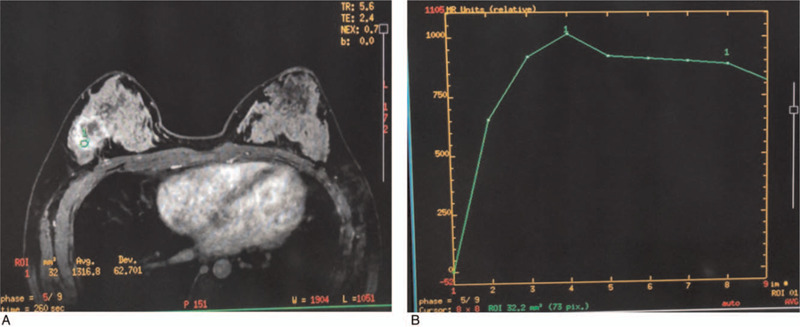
MRI imaging before the 5th cycle of neoadjuvant chemotherapy. (A) A 3.1-cm mass in right breast (BI-RADS 6); (B) time-signal curve demonstrated outflow type.
